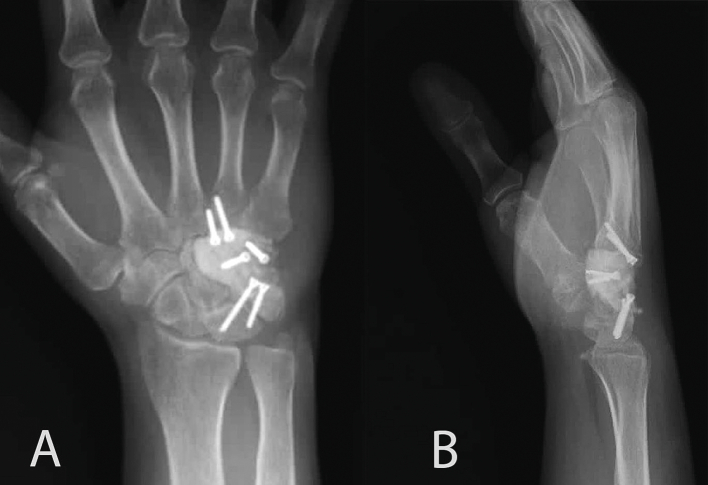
(a) Postoperative anteroposterior and (b) sagittal radiographs of the wrist showing final reconstruction with polymethylmethacrylate cement (PMMA) and a four-corner wrist arthrodesis mini plate.
